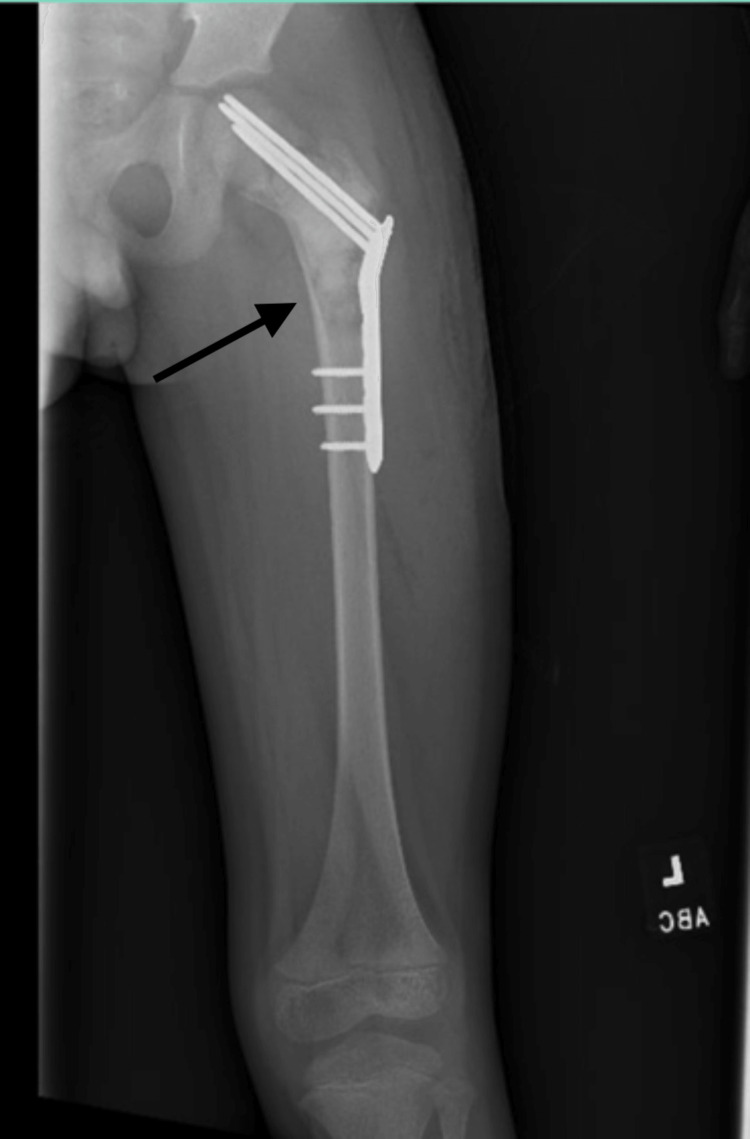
Radiograph after open reduction and internal fixation of a pathologic fracture and curettage of an aneurysmal bone cyst
